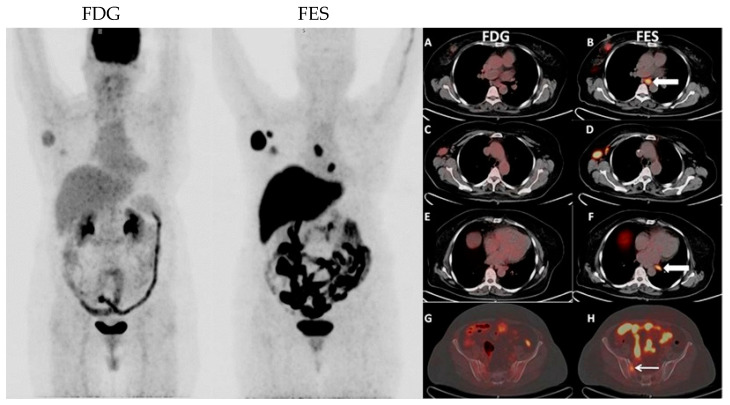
Well-differentiated estrogen receptor (ER)-positive breast tumor with significantly less radiotracer uptake on ^18^F-FDG PET/CT than on 16α-[^18^F]fluoroestradiol (FES) PET/CT, with FES showing more sites of ER-positive disease. The images show FES-avid mediastinal lymph nodes and right iliac bone lesion (B,D,F,H; white arrows in FES images) with significantly less ^18^F-FDG uptake (A,C,E,G).
